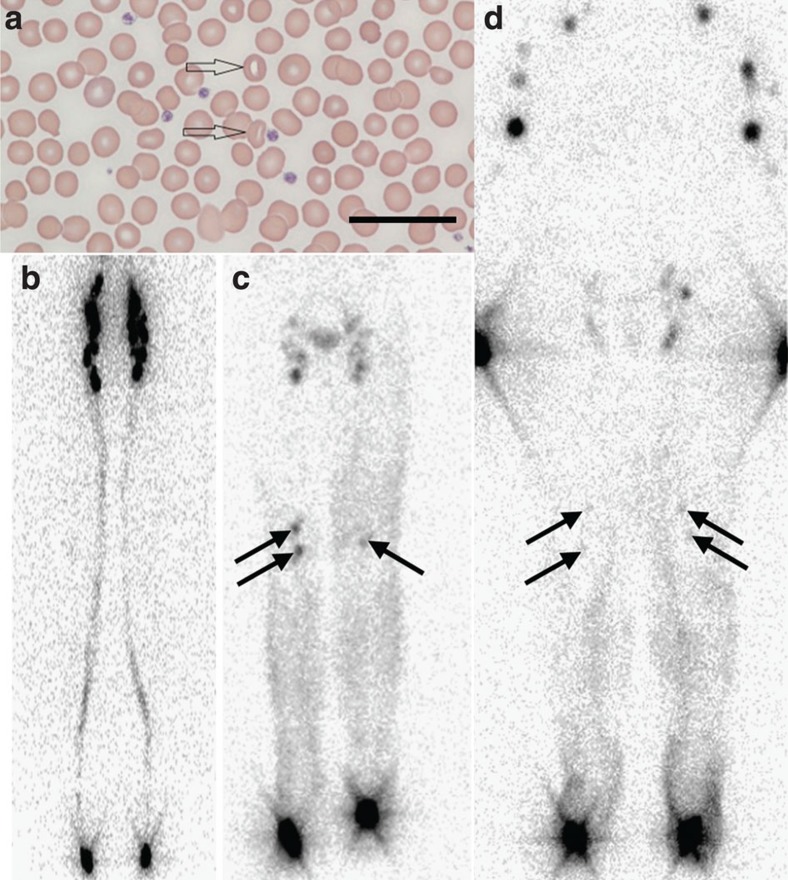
Blood analysis and lymphoscintigraphy. (a) Blood film showing occasional stomatocytes (arrows) in GLD1:II.2. Scale bar, 20 μm. (b) Lower limb lymphoscintigraphy in an unaffected subject showing symmetrical migration of radionuclide through discrete lymph vessels 2 h after injection. (c) Lower limb lymphoscintigraphy of GLD4:II.3 and (d) four limb lymphoscintigraphy in GLD1:II.3. The three lymph scans have been aligned so that injection sites in the feet are all at the bottom of the panel with the groin area all at the same level at the top of b and c. The injection sites in the hands in d are the dark areas on either side of the groin region and axillary lymph nodes are visible at the top of d. The lymph scans of the two patients (c,d) show distinctive changes with poor uptake of tracer in the groin and axillae at 2 h, with evidence of rerouting in the lower limbs (seen as the dark shading of the lower limb). Popliteal lymph nodes (arrows) show prominent uptake of tracer, which is unusual and represents deep rerouting of the interstitial fluid.
